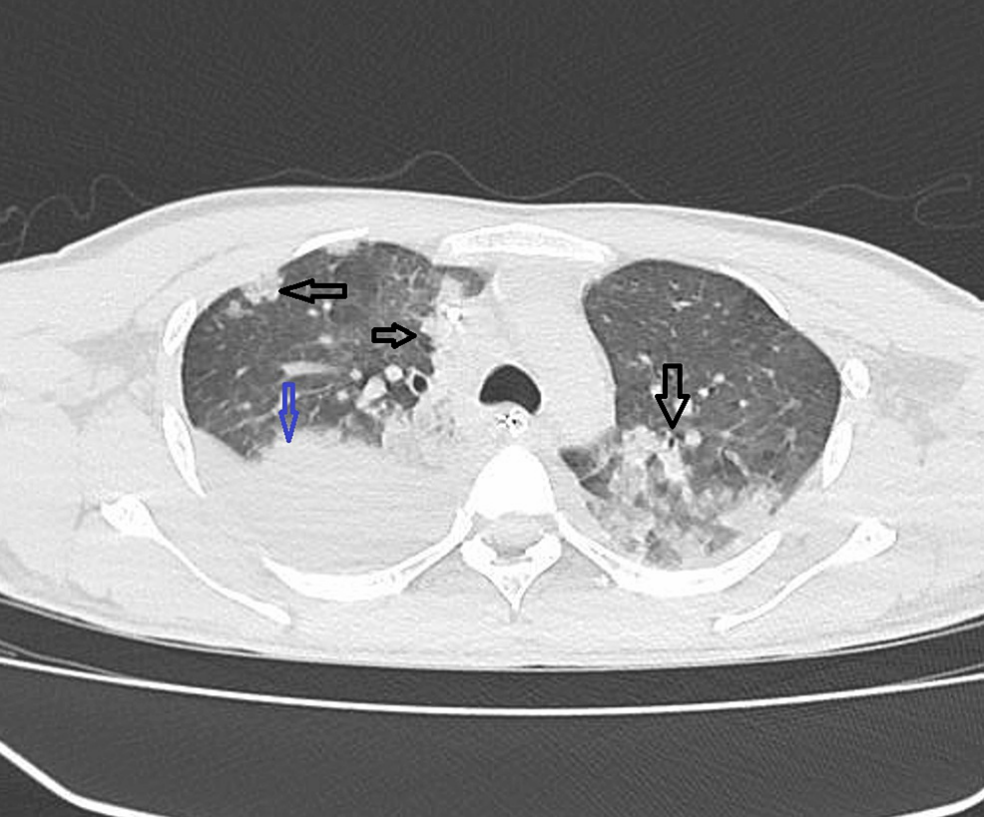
CT chest showing bilateral pulmonary infiltrates and right-sided effusion. The blue arrow shows right-side effusion and the black arrows show bilateral infiltrates.
